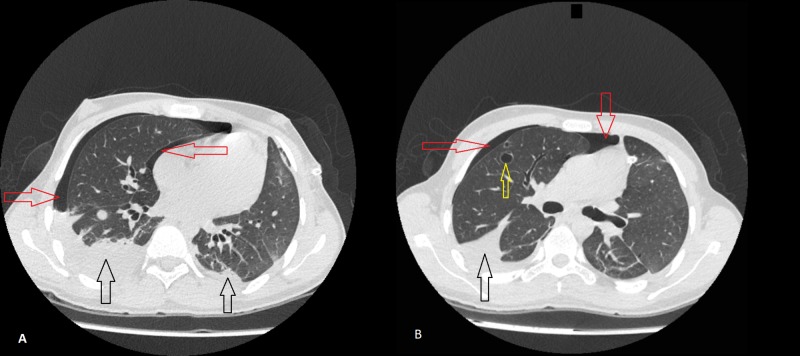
Computed tomography (CT) scan of the chest without contrast. Image A: Red arrows point toward pneumothorax in the right lung; black arrows point toward bilateral pleural effusions. Image B: Red arrows point toward pneumothorax in the right lung; black arrow points toward a pleural effusion; yellow arrow points toward a cystic lesion (1.8 cm) in the upper lobe of the right lung.
